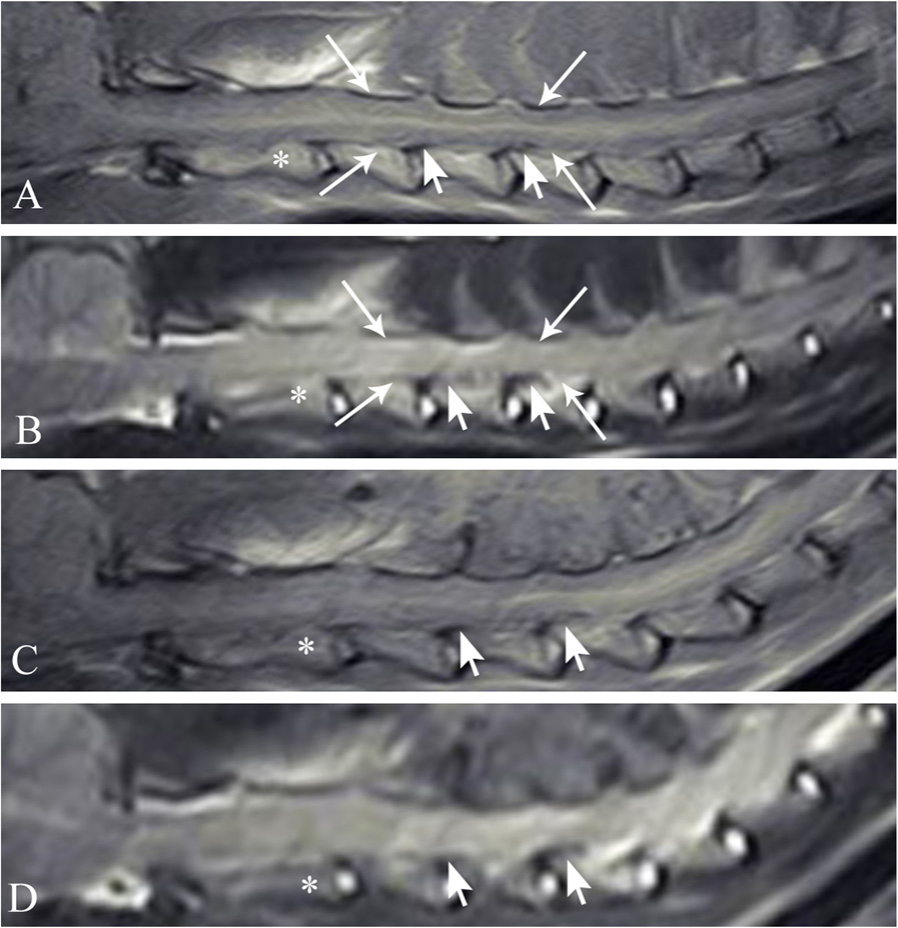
Sagittal planes of magnetic resonance images T1-weighted (A), T2-weighted (B) before surgery and T1-weighted (C), T2-weighted (D) after surgery. Intradural extramedullary/extradural hypointensity in the vertebral canal (pointer arrows) in T1-weighted and T2-weighted images corresponds to a radiopaque line evident in the lateral radiograph at the level of C3-5. The arrows depict stenosis of the vertebral canal and flattening/compression of the spinal cord; the asterisk denotes the C2 vertebra.
